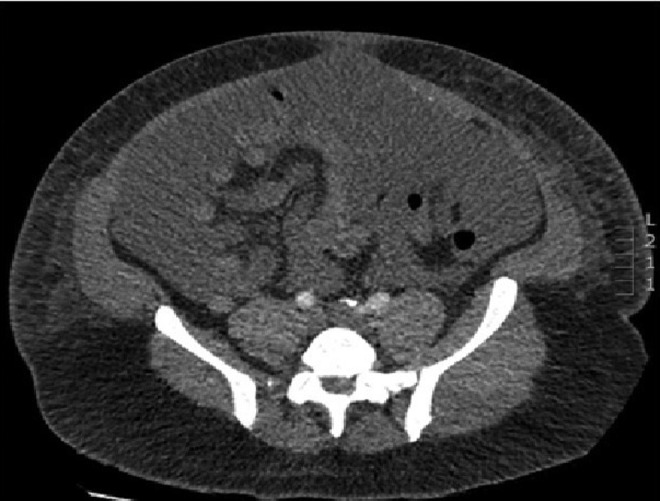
Abdominal CT scan shows massive ascites.
